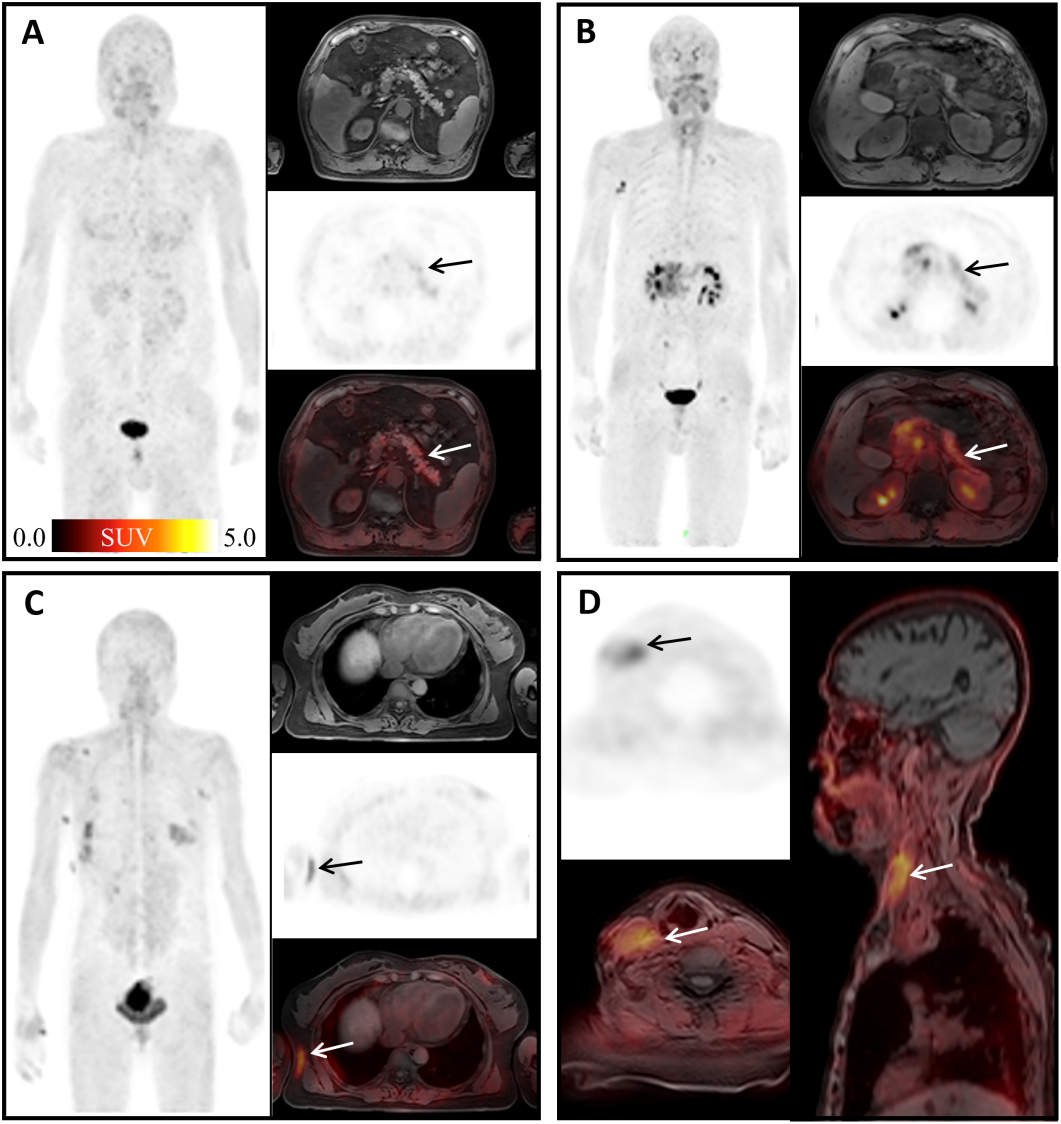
Physiological uptake of ^68^Ga-FAPI-04. (A) A 67-year-old man with mucinous adenocarcinoma of the right lung 1 year after surgery; arrows show pancreatic diffuse inflammatory uptake, SUV_max_ =4.66. (B) A 63-year-old man diagnosed with duodenal malignancy; arrows show obstructive pancreatitis, SUV_max_ = 18.11. (C) A 49-year-old woman, 3 months after surgery for early microinfiltrating adenocarcinoma of the right middle lobe and 2 months after surgery for left breast fibroma; arrows show surgical scar on the right chest with SUV_max_ =3.89. (D) An 83-year-old woman diagnosed with gastric cancer 6 months ago; arrows show deep vein catheterization area of the right neck with SUV_max_ =4.56.
